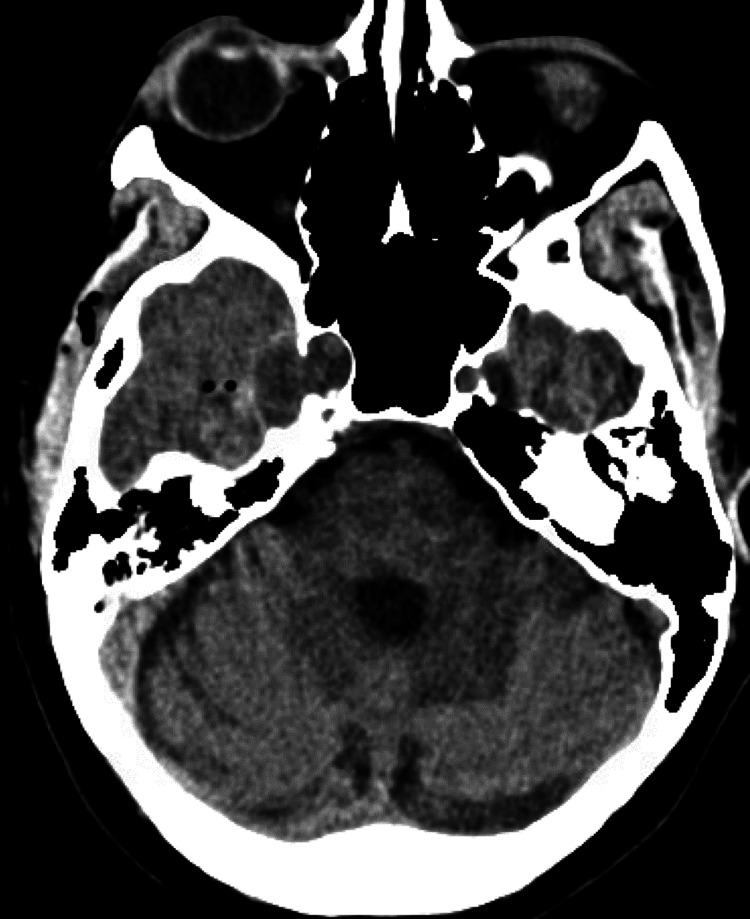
Postoperative imaging A non-contrast CT scan performed the morning following surgery.
